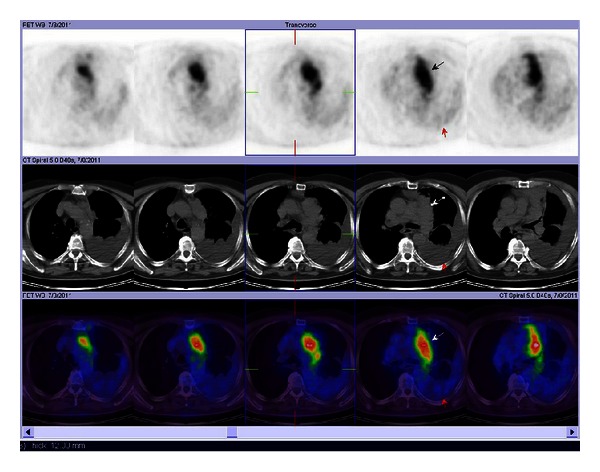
PET/CT scan shows intense and homogenous hypermetabolic activity at the upper anterior paramediastinal region (SUV: 7) and there was hypermetabolic lesions at the left basal pleura, which was compatible with metastases.
